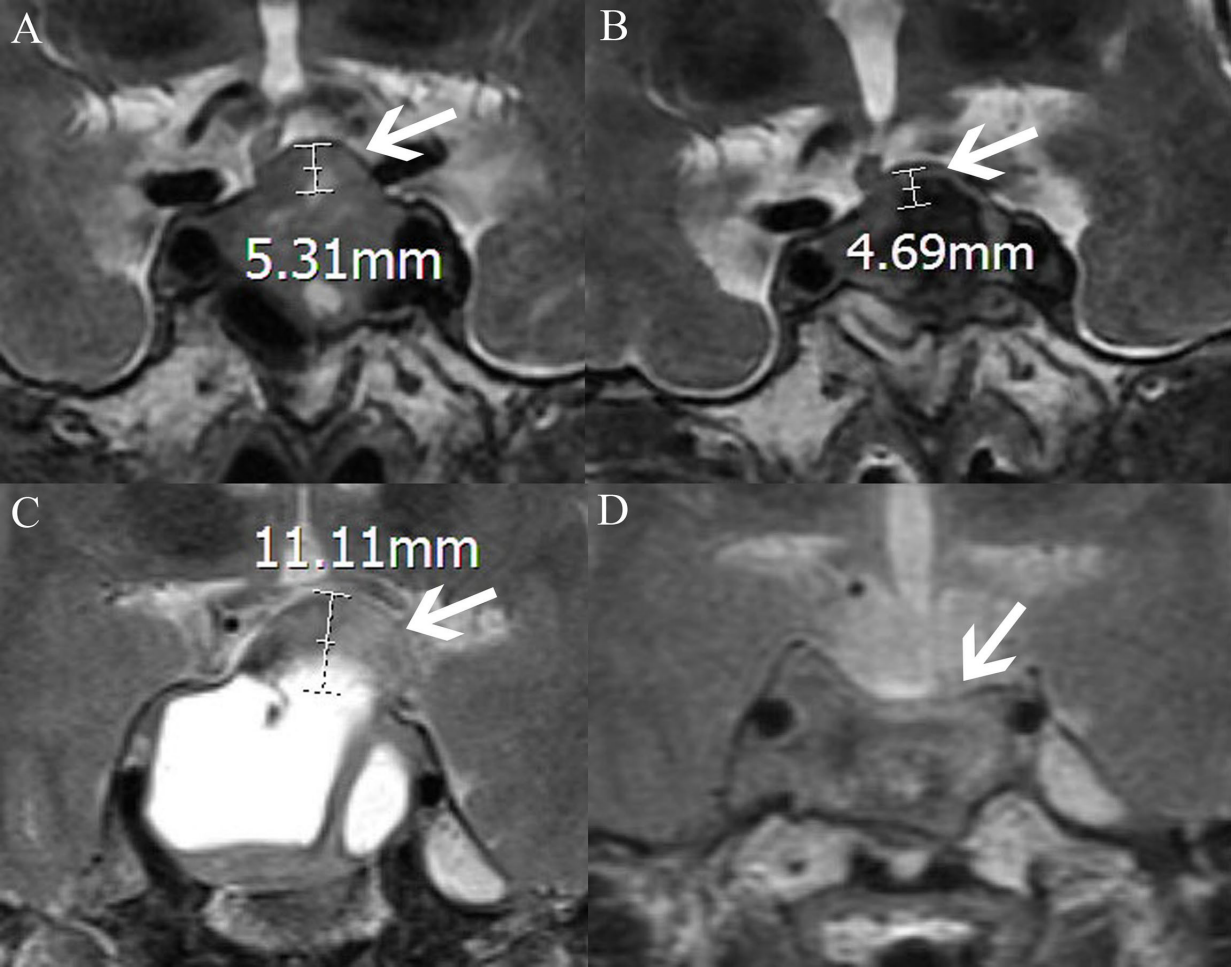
MRI-T2WI shows the measurement of the elevation of the diaphragm sellae (the distance between the plane where the diaphragm sellae starts to rise and the plane of the highest point of the diaphragm sellae; the white arrow points to the diaphragm sellae, AB and CD, respectively, represent the same patient). (A) The elevation of the diaphragm sellae before surgery is 5.31 mm. (B) The elevation of the diaphragm sellae after surgery is 4.69 mm. The diaphragma sellae sinking depth is the difference between the two values (5.31–4.69 mm). (C) The elevation of the diaphragm sellae before surgery is 11.11 mm. (D) The elevation of the diaphragm sellae after surgery is 0 mm. The diaphragma sellae sinking depth is the difference between the two values (11.11–0 mm).
